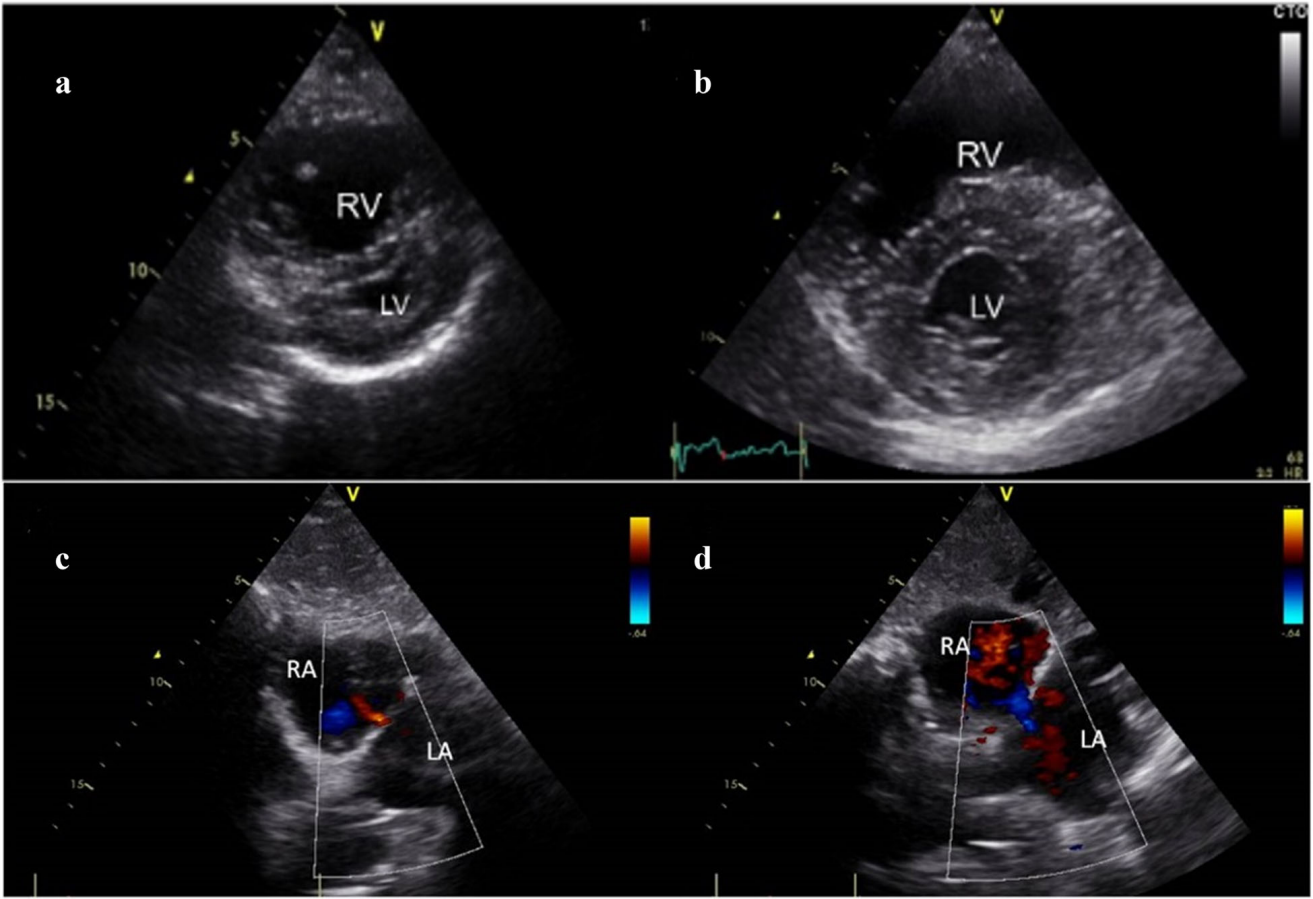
a, b Short-axis transthoracic echocardiographic view demonstrating the positive remodelling of the *right ventricle* (RV) 2 years after the atrial septostomy and the initiation of subcutaneous treprostinil. LV = *left ventricle*
